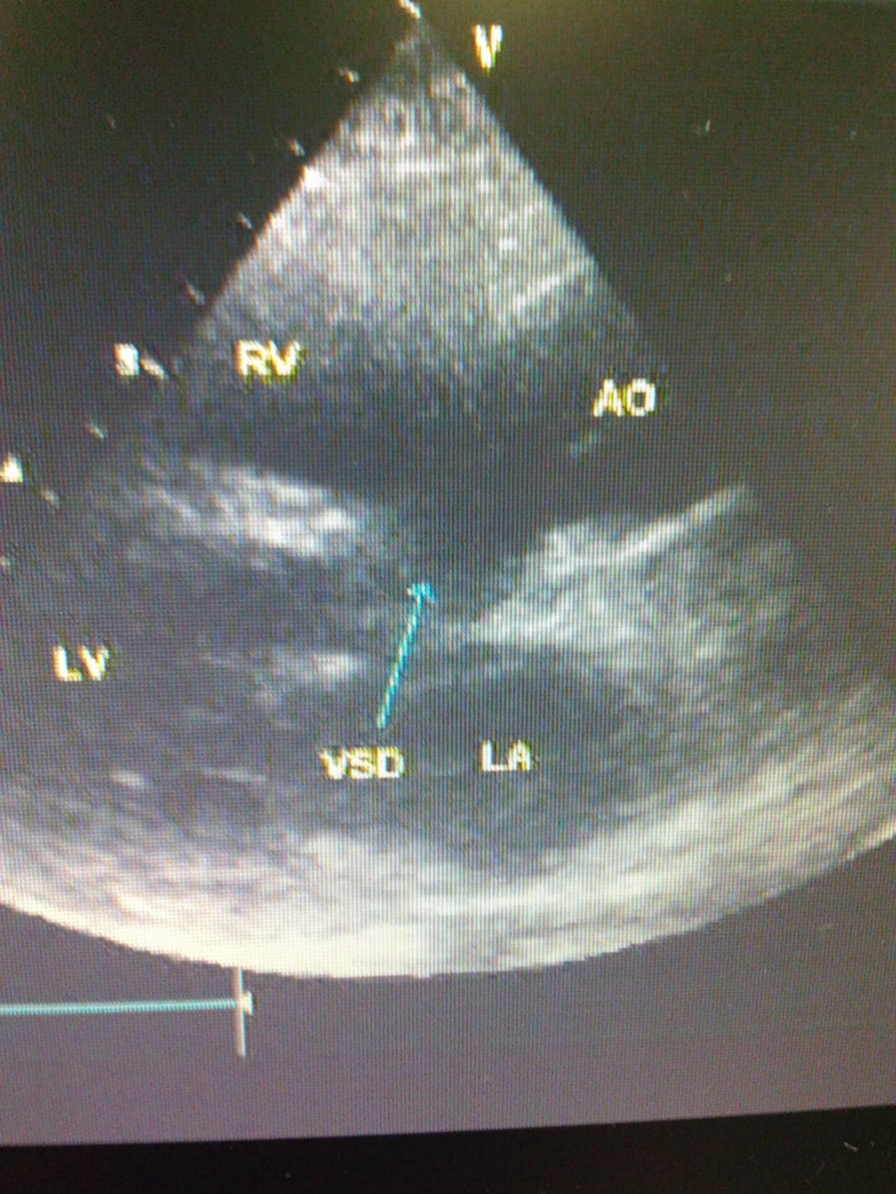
Echocardiogram of the pregnant woman. Cardiac function: EF was 58 %, FS was 30 %. Dimensional and M echocardiograph: enlargement of right atria and ventricular (RA 55 cm, RV 30 cm); the aorta and pulmonary artery were parallel without enlargement; the aorta ridded on ventricular septum (80 % was from right ventricular); the defect of ventricular septum was 26 mm which was under aorta; there was absence of fibrous joint between anterior mitral valve leaflet and the posterior wall of aorta. Color Doppler ultrasound: there was a left to right shunt between ventricularis was detected (V_max_ was 1.6 m/s)
